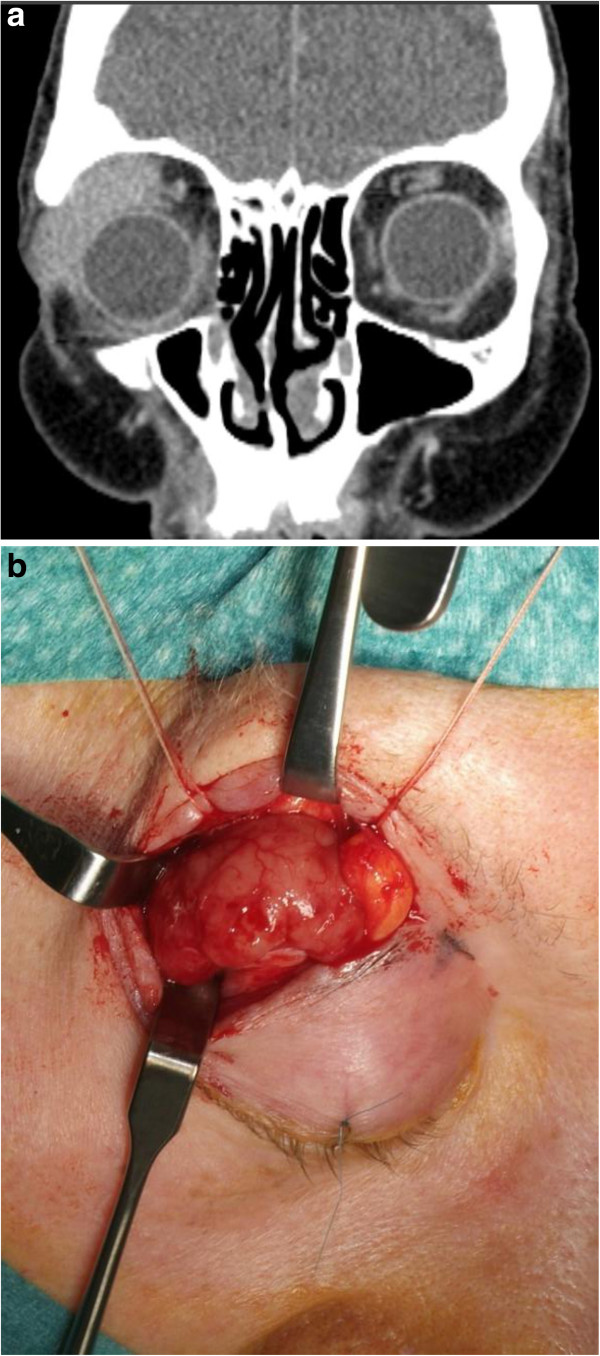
A patient with involvement of the lacrimal gland received complete surgical removal as primary treatment. (a) Coronal computed tomography scan showing a tumor lesion in the upper right orbit of a 77-year-old woman. (b) The tumor was exposed using a blepharoplasty approach and completely removed together with the lacrimal gland.
